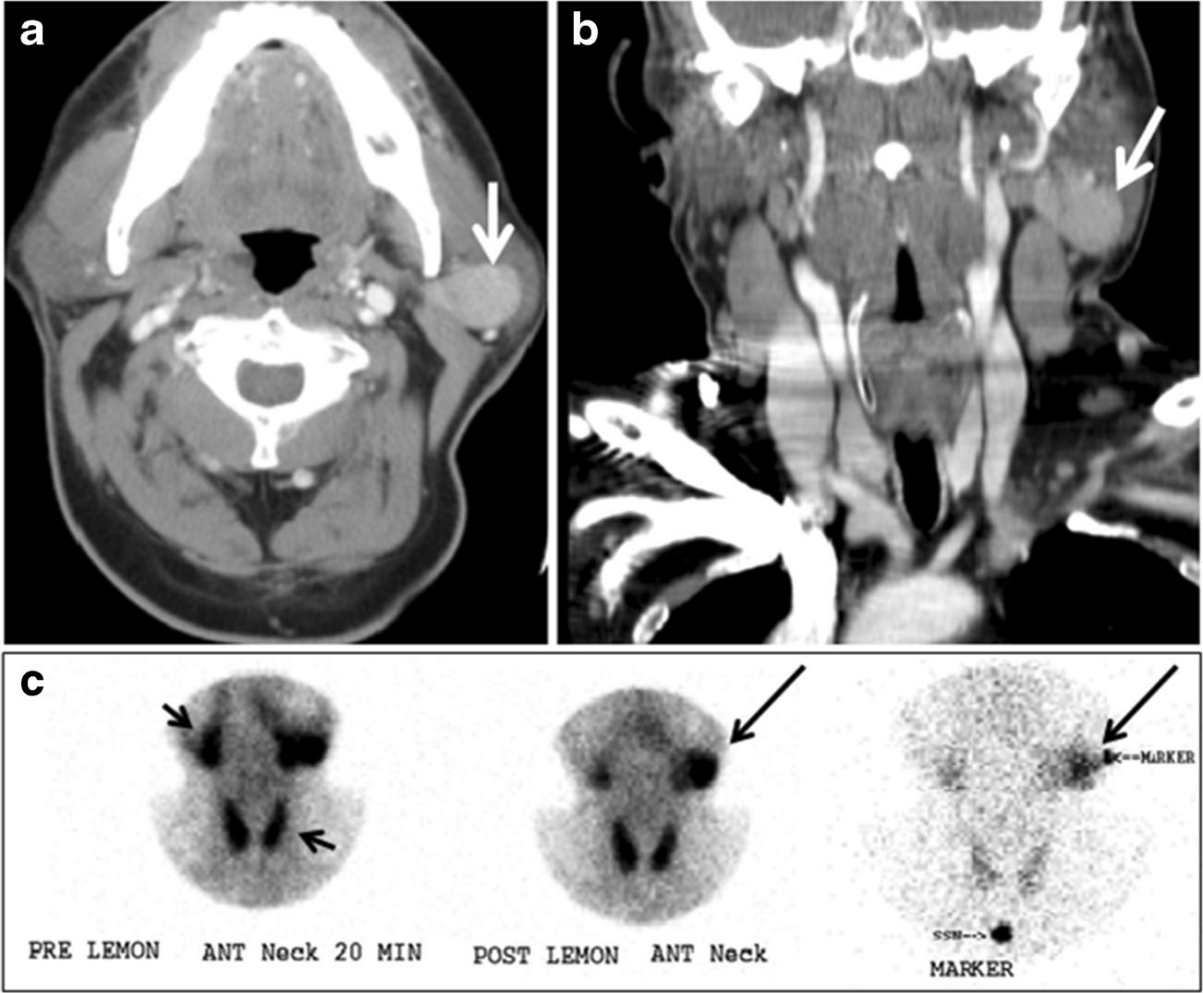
Ectopic thyroid on the left parotid gland with a palpable left parotid mass in a 69-year-old male patient. a, b Axial and coronal enhanced neck CT scan demonstrates well-defined homogeneous enhancing mass (*white arrows*) within the left parotid gland with preserved surrounding fat planes. It also shows a normal thyroid in normal position in the lower neck. c Image taken 20 minutes after 5 mCi injected Tc99m-Pertechnetate shows normal thyroid uptake of tracer and physiological uptake in the salivary glands (*short black arrow*). There is a distinct focus of abnormal tracer accumulation in the left parotid/submandibular region. Patient was given lemon juice with evident normal washout from the salivary glands and relative retention by this abnormal focus (*long black arrow*)
